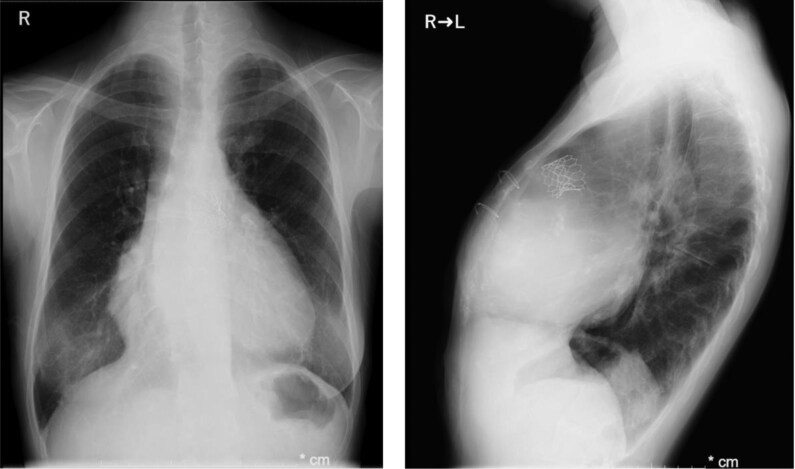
Postoperative Day 4 chest radiographs (frontal and lateral views) showing a cardiothoracic ratio of 62.6%.
